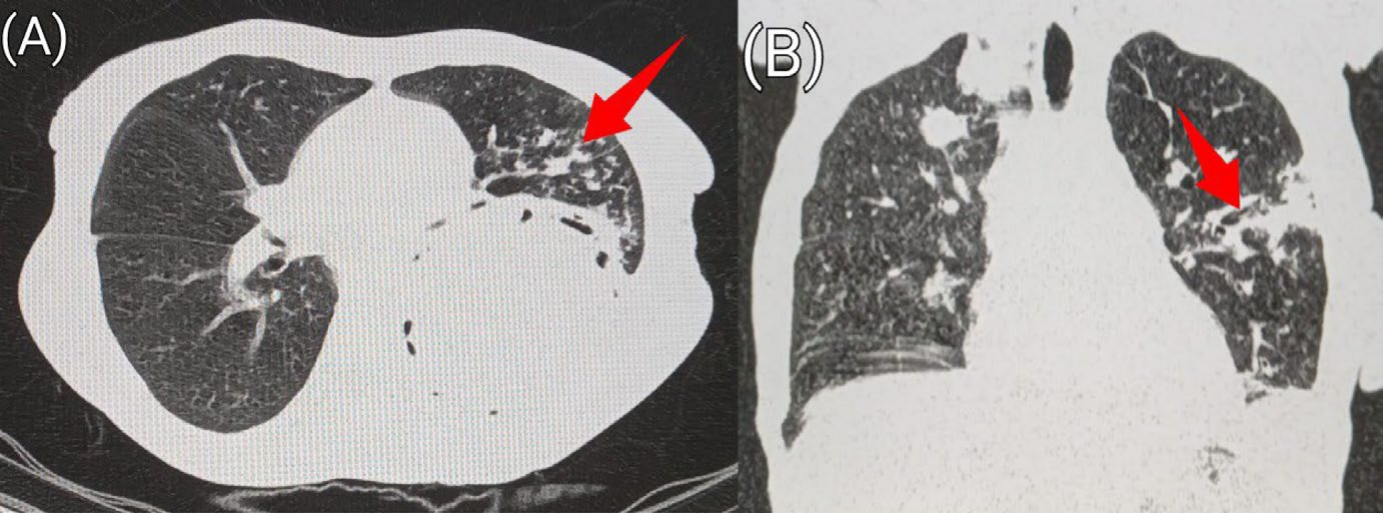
Lung window of pre‐contrast axial (A) and sagittal (B) CT sections of the chest showing fibrotic changes with interstitial thickening (red arrow) in the left lower lobe.
